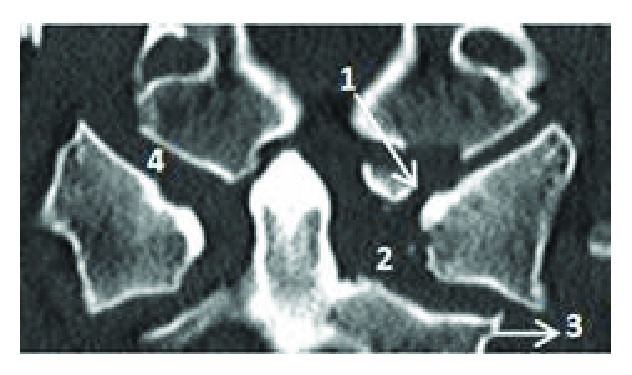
Cervical spine CT scan showing type III left occipital condyle fracture (1), asymmetric left odontoid-lateral mass widening (2), lateral subluxation of bilateral C1 lateral masses (3), and widening of the right atlantooccipital joint (4).
